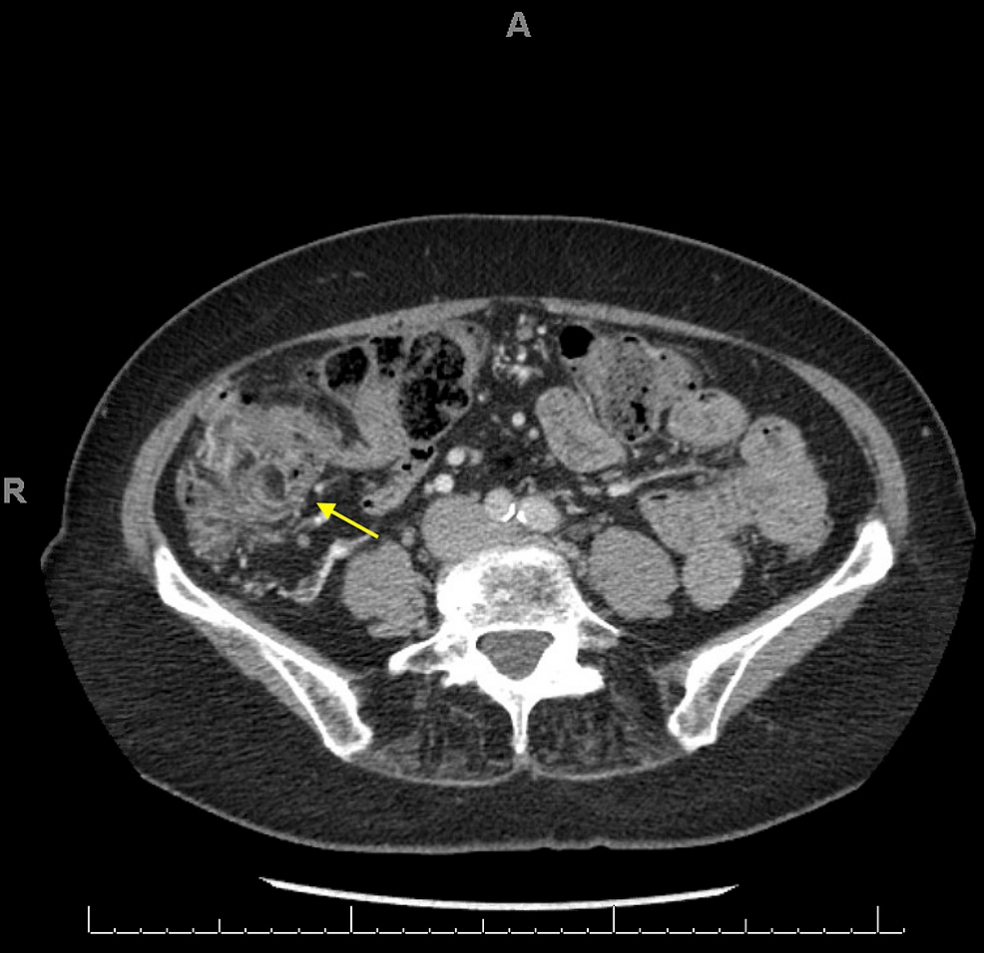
CT abdomen and pelvis showing mucosal thickening and hyperenhancement with slight vascular engorgement in the cecum and ascending colon
